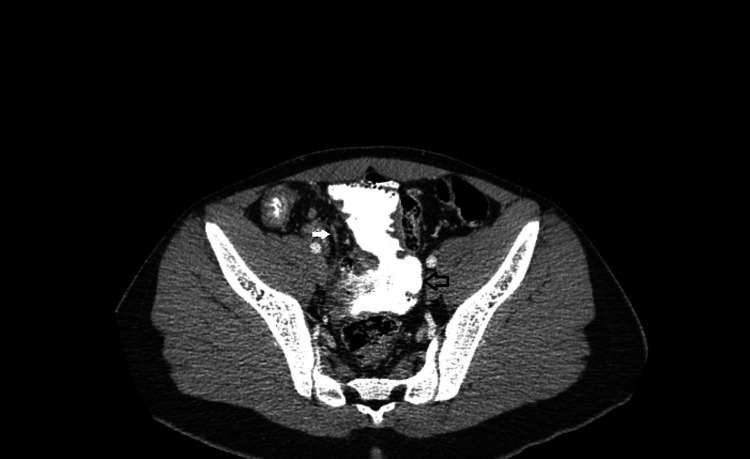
Enhanced CT with oral contrast, axial view showing the appendix (white arrow) and the cecum
